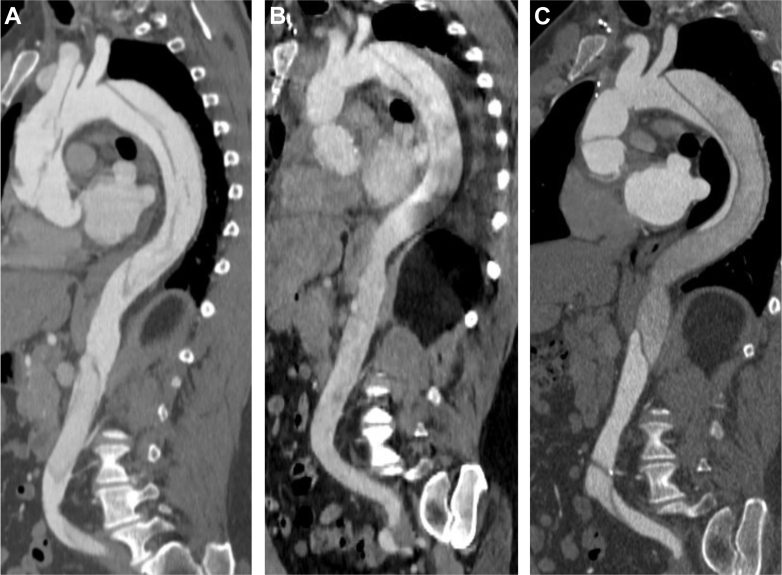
Computed Tomography Imaging of a 56-Year-Old Man With Aortic Dissection (A) Initial presentation shows an extensive thoracic and abdominal AD originating in the ascending aorta, sparing the aortic root, coronary arteries, and major branches, with super mesenteric artery perfused by the false lumen. (B) Postsurgical repair with a 32-mm Hemashield graft in the ascending aorta, aortic valve resuspension, and aortic arch reconstruction, leaving residual descending AD unrepaired. (C) Sixteen months after surgery, follow-up imaging shows stable graft position and unchanged residual descending AD.
